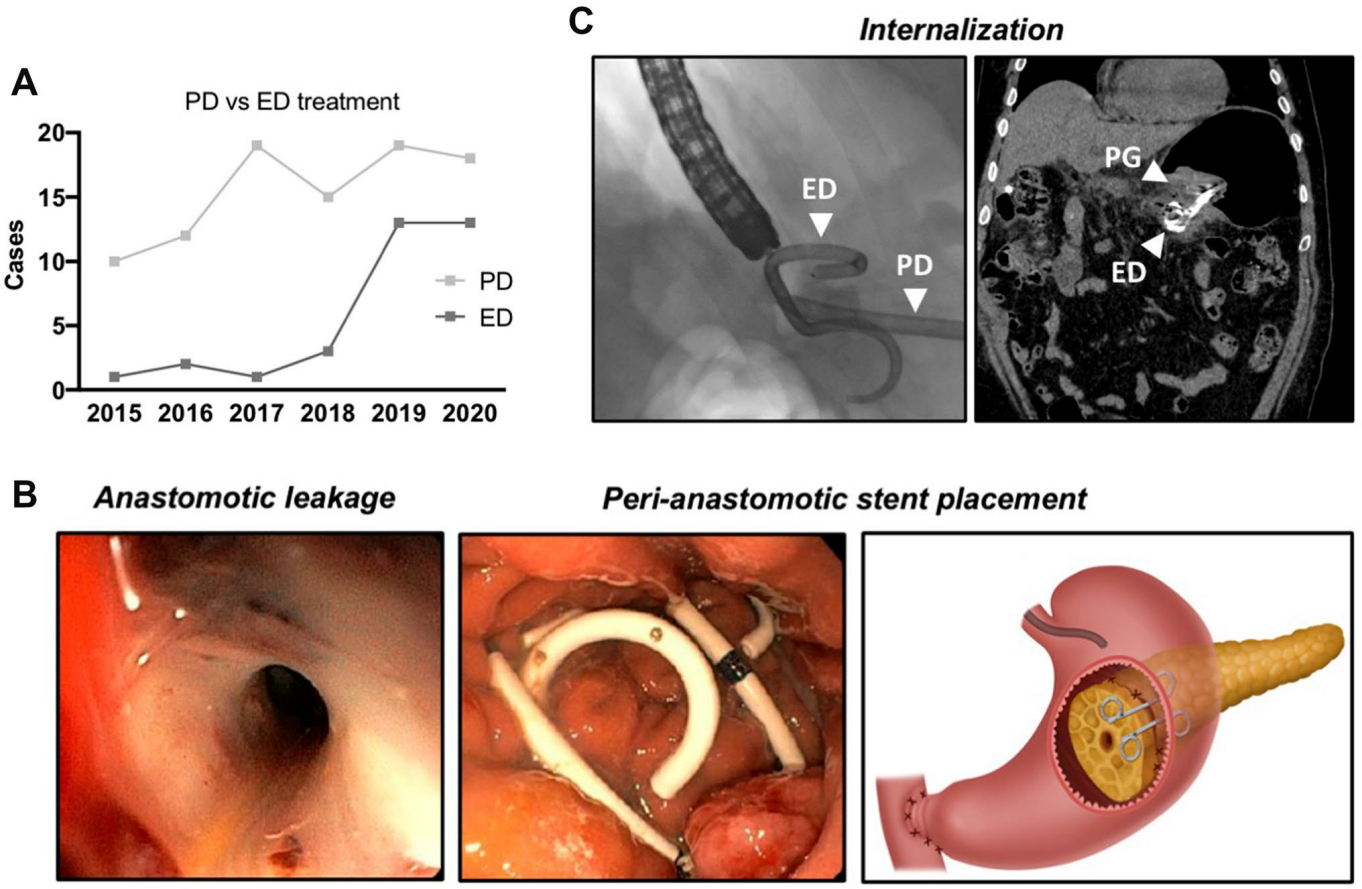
A The number of endoscopic treatments over the years 2015–2020. B Representative pictures during endoscopic intervention showing large dehiscence of the PG with subsequent placement of two plastic pigtail stents. C A guidewire and the tip of the percutaneous drainage (PD) helped to correctly place the peri-anastomotic pigtail stents (ED). Representative post-interventional CT-scan shows correct stent placement and internal drainage of peri-anastomotic fluid collection at the pancreato-gastrostomy (PG)
